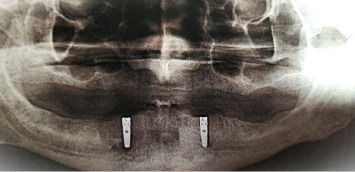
X-ray showing two implants inserted within the interforaminal region of the patient's mandible.
